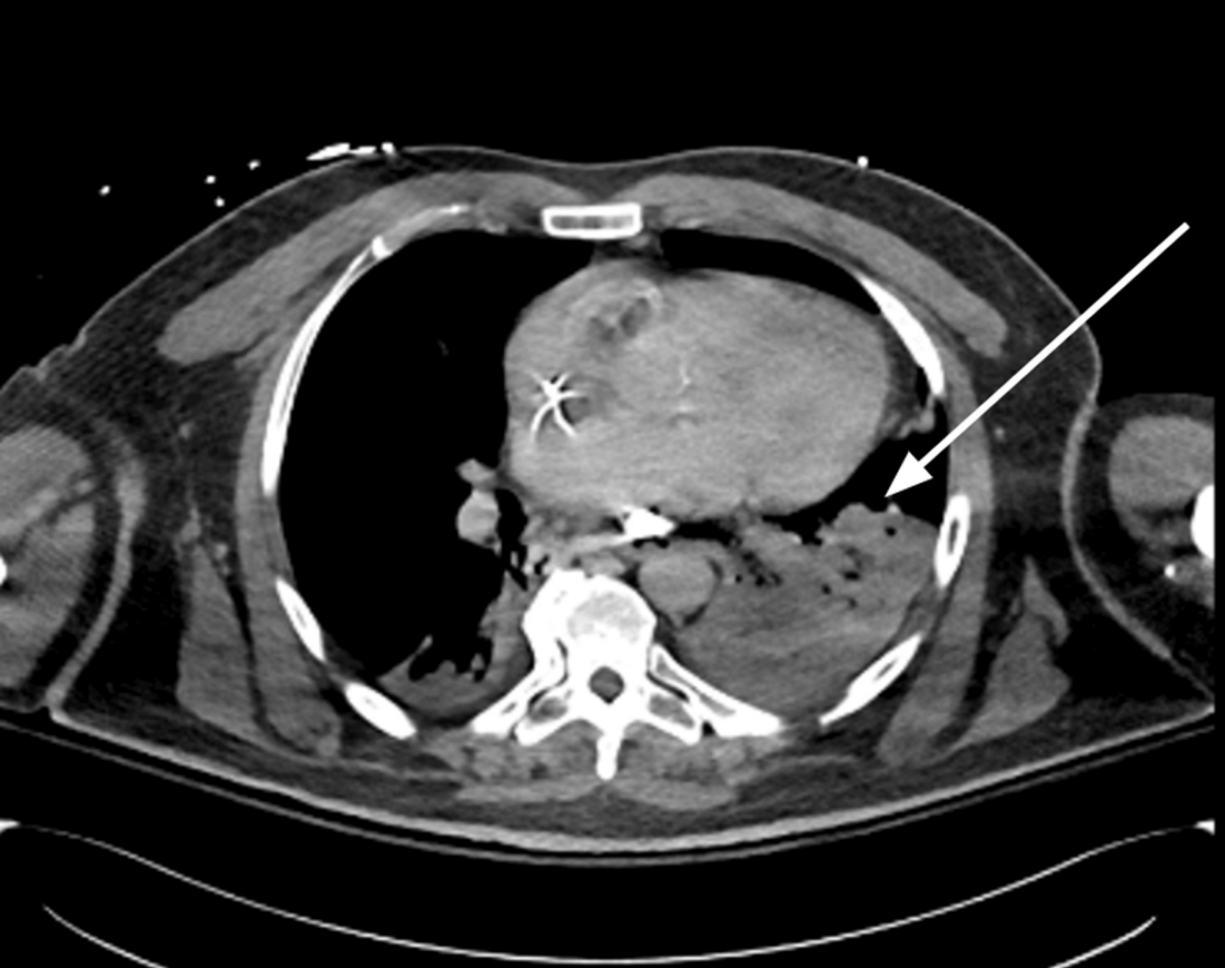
CT chest with IV contrast showing the Blakemore catheter has perforated the distal esophagus and is malpositioned and insufflated in the left lower lobe (LLL) with associated small left pneumothorax and complete LLL consolidation
